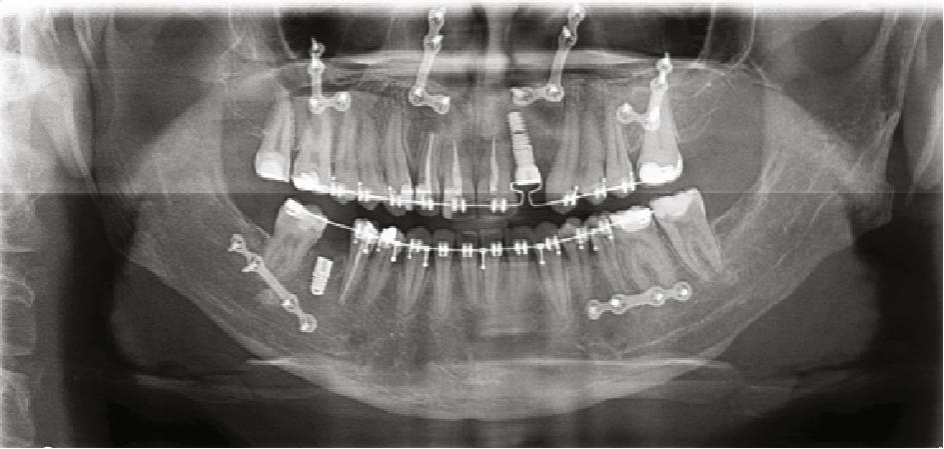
The panoramic radiograph of the patient after the implant surgery.
